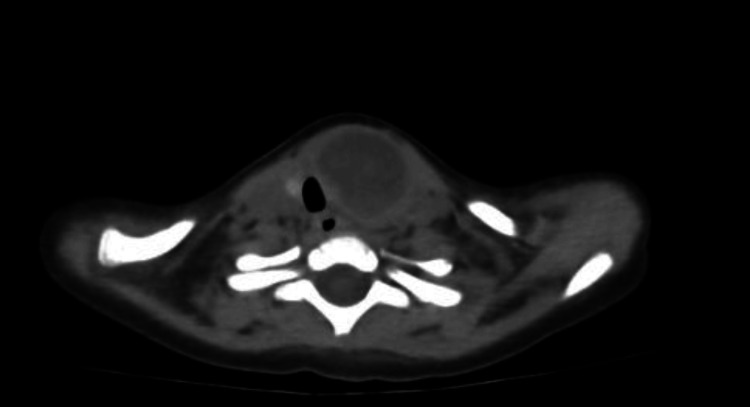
Cervical CT scan: axial view A cervical CT scan revealed a collection that caused the left deviation of the trachea and extended into the retropharyngeal space. CT: computed tomography
